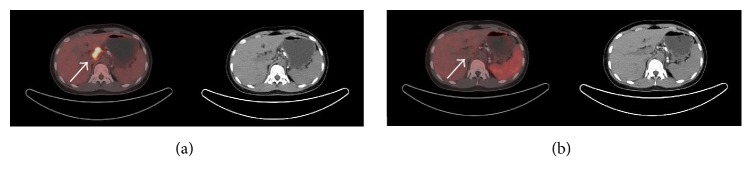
^18^F-FDG (a) and ^18^F-Alfatide II (b) PET/CT images of a lymph node tuberculosis patient. Lymph nodes tuberculosis lesions showed intense ^18^F-FDG uptake and no ^18^F-Alfatide II uptake. The white arrows indicate tuberculosis lesions in porta hepatis lymph nodes.
